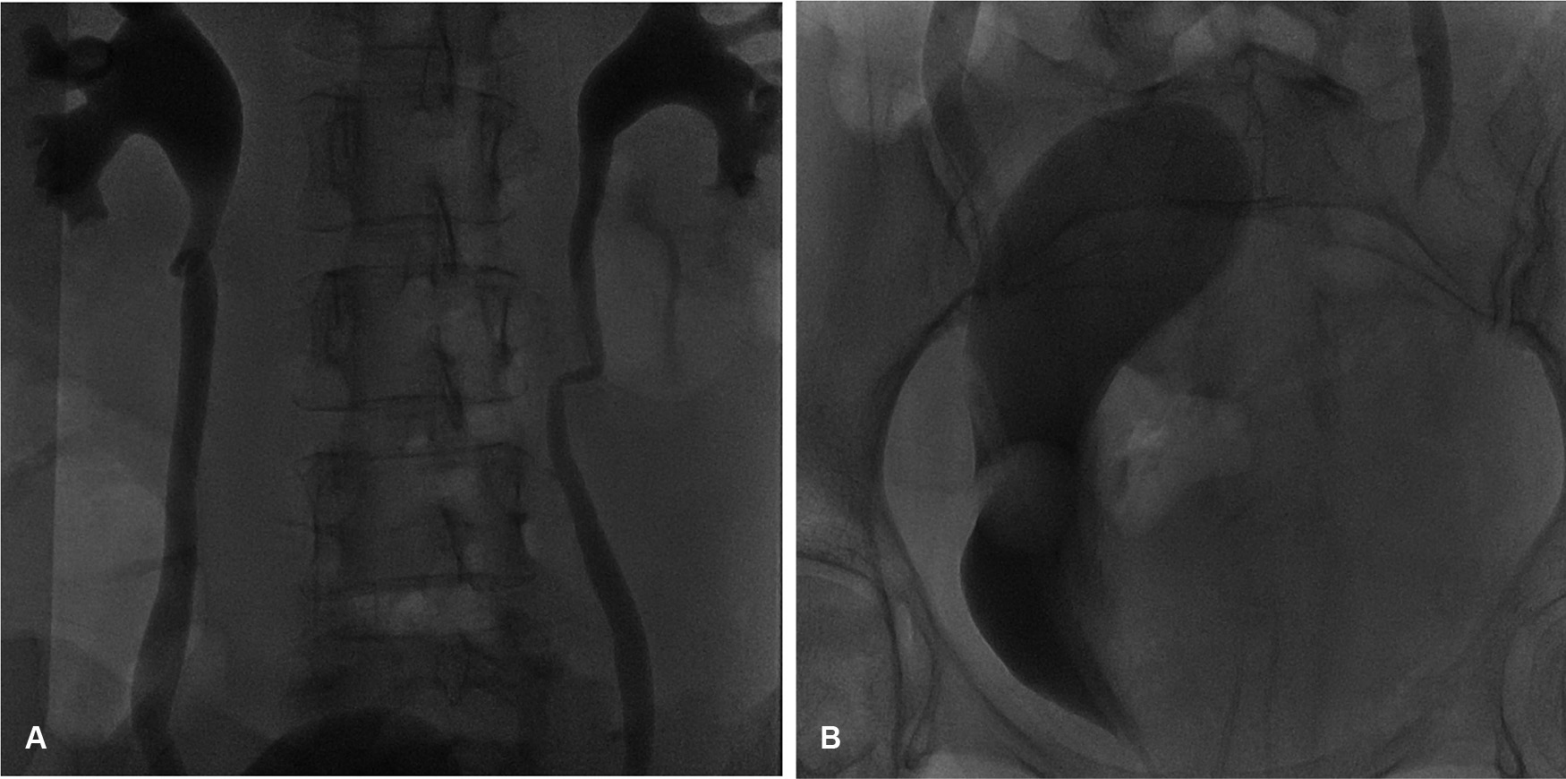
X-ray angiography showing (A) bilateral dilation of the pyelocaliceal system and (B) bladder displaced to the right.
